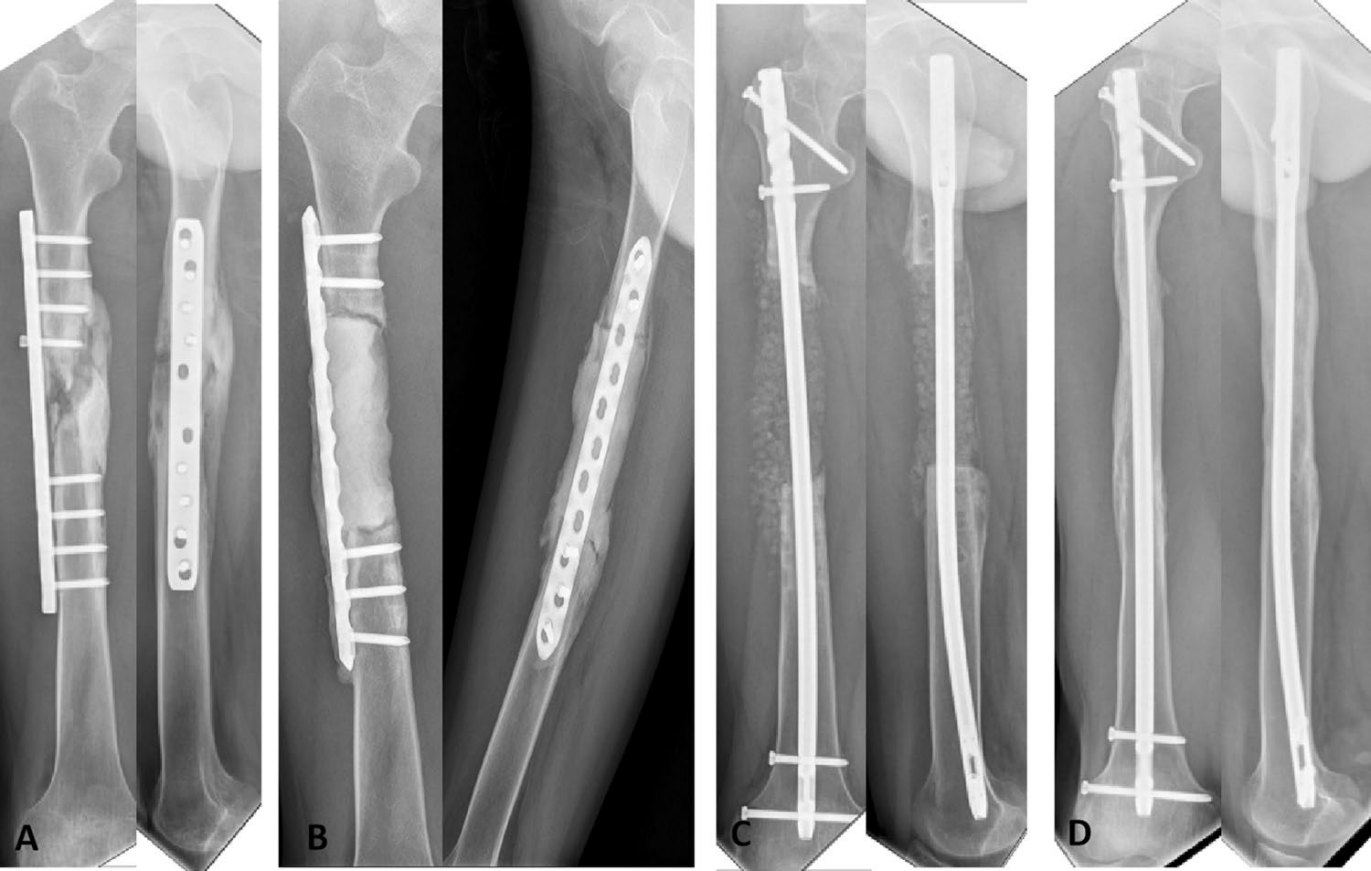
Typical case: Male, 17Y, repeated draining fistula of the right femur for 8 months, bone defect 10.5 cm. (A) Preoperative X rays; (B) 10.5 cm bone defects were implanted with PMMA cements; (C) X-rays of the bone defects 1 month after grafting; (D) X-rays showed bone union after 18 months.
